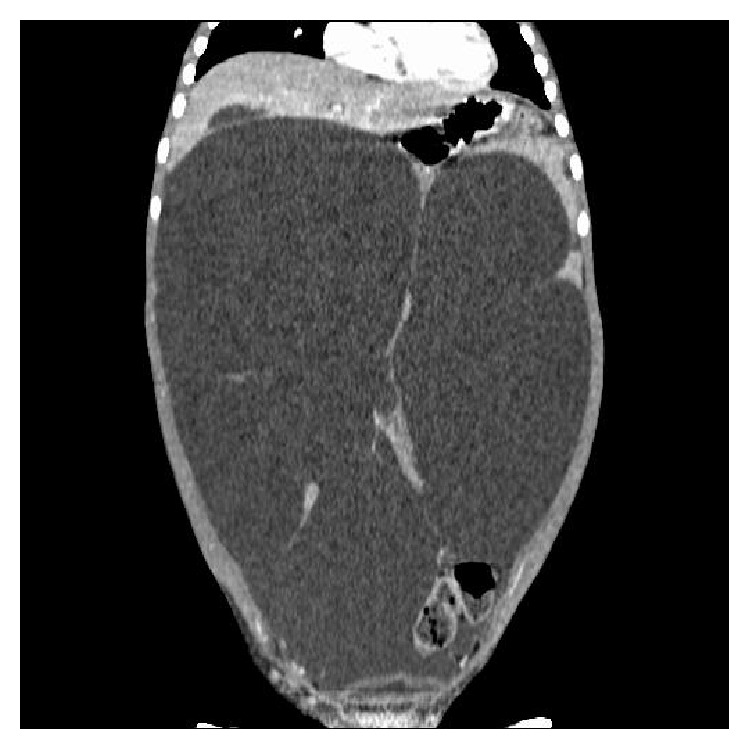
CT scan of abdomen and pelvis showing a giant multiloculated cystic mass occupying the entire abdomen with mass effect on the surrounding structures.
